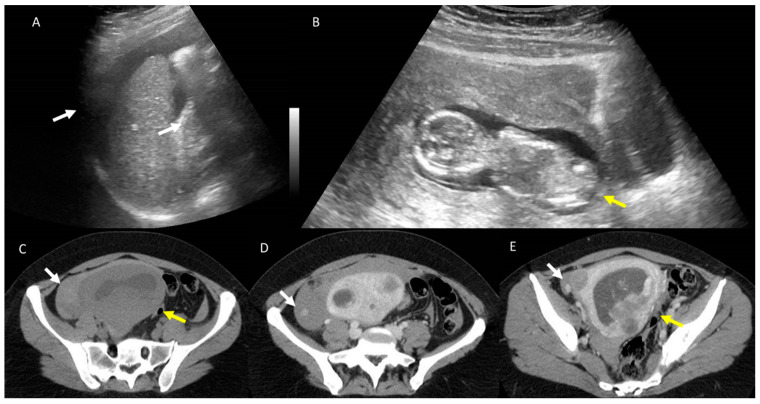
Polytraumatized (crash accident) pregnant woman (yellow arrows) admitted to emergency room. Fast US (A and B) shows abdominal free fluid. Unenhanced CT (C), white arrow shows intraabdominal blood with active bleeding (white arrows in D,E) after contrast medium administration.
